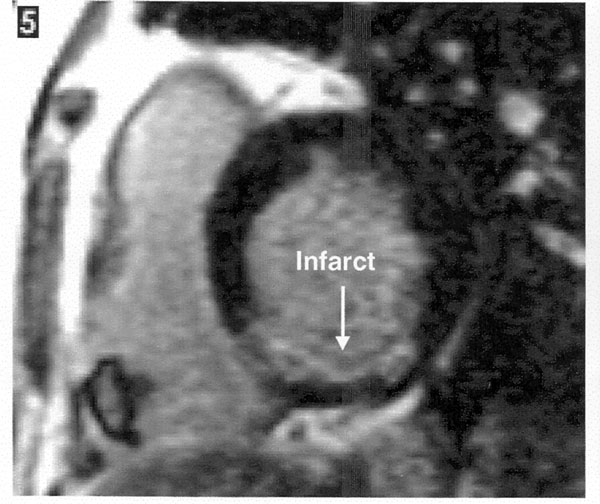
Cardiac magnetic resonance imaging showing the subendocardial infarction of the right ventricle.
